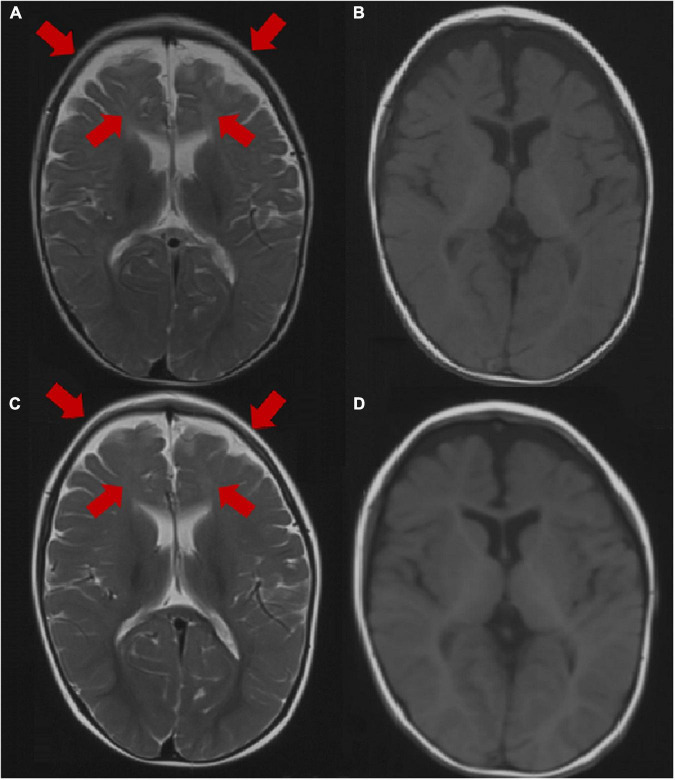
Brain MRI image of patient 3 at the age of 1 year. (A,B) Axial images (T1WI and T2WI) showing dysplasia of the frontal and temporal cortex and delayed myelination. Brain MRI image of patient 3 at the age of 2 years. (C,D) Axial images (T1WI, T2WI) showing dysplasia of the frontal cortex and delayed myelination. The arrow points to the lesion.
